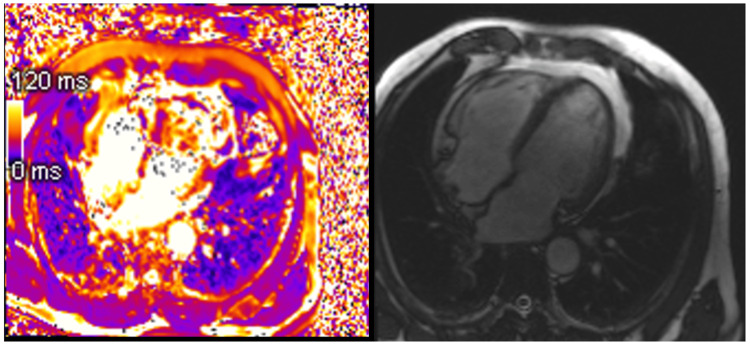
Cardiac MRI showing no evidence of cardiac hydatid involvement MRI: magnetic resonance imaging
